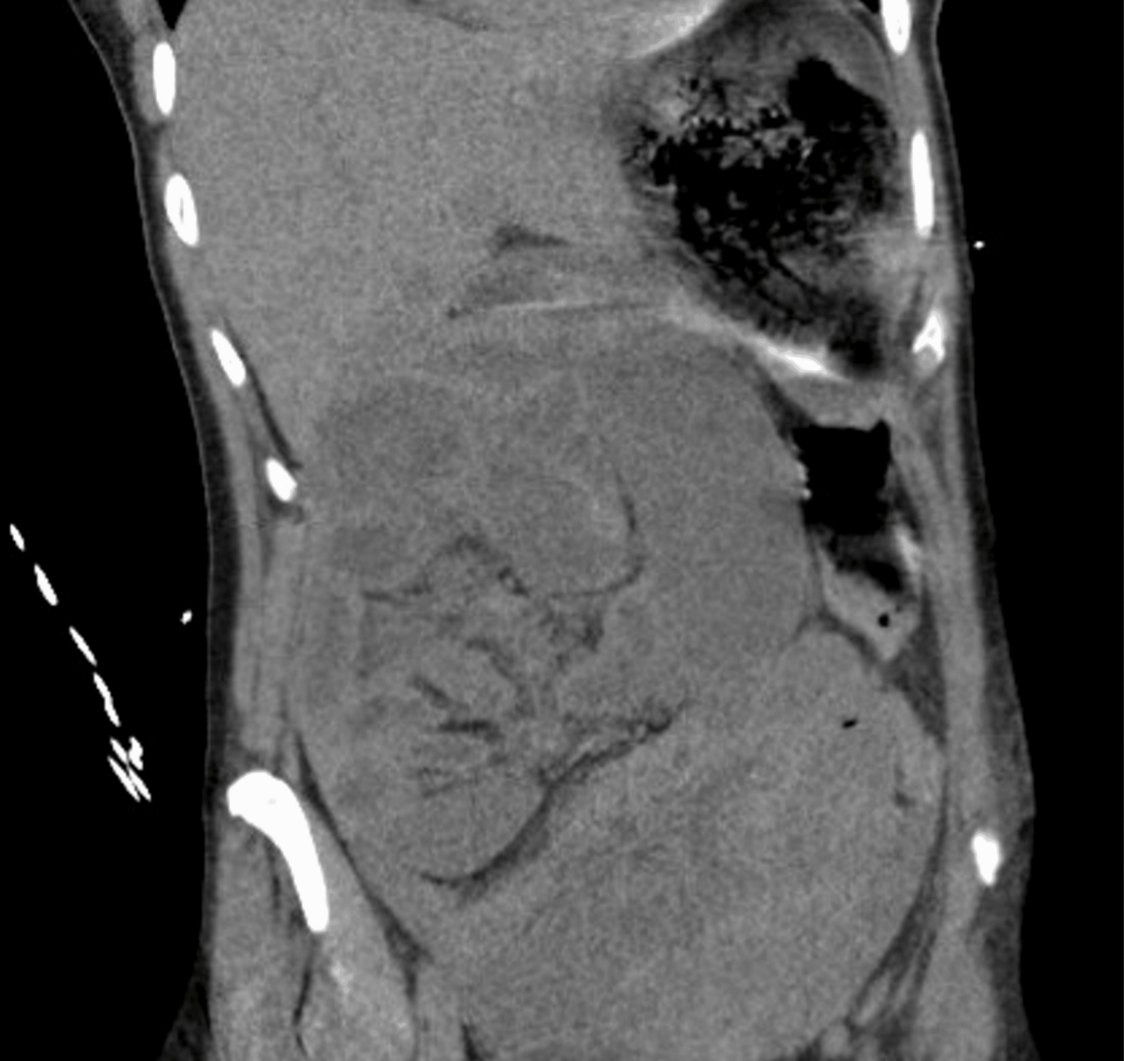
CT abdomen and pelvis without IV contrast (coronal) Coronal view of the CT abdomen and pelvis, revealing dilated small bowel all on the right side of the abdomen, and a lack of cecum in the right lower quadrant of the abdomen.
